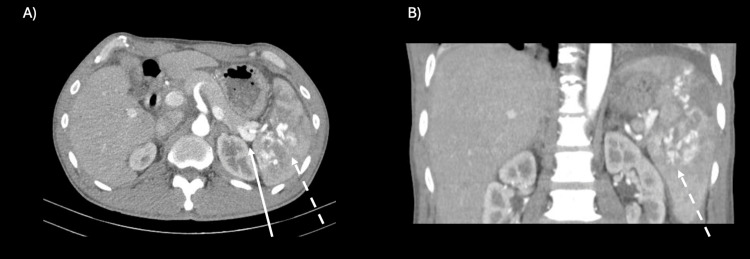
Contrast-enhanced CT on day five of hospitalization (A) shows splenic injury in horizontal view in the early phase of contrast-enhanced CT, with pseudoaneurysm (white dotted arrow) and early depiction of splenic vein (white arrow), suggesting a traumatic AVF via a pseudoaneurysm. In (B), the coronal view of the early phase of contrast-enhanced CT shows the same site as in (A), indicating multiple pseudoaneurysms (white dotted arrow). CT: computed tomography; AVF: arteriovenous fistula
